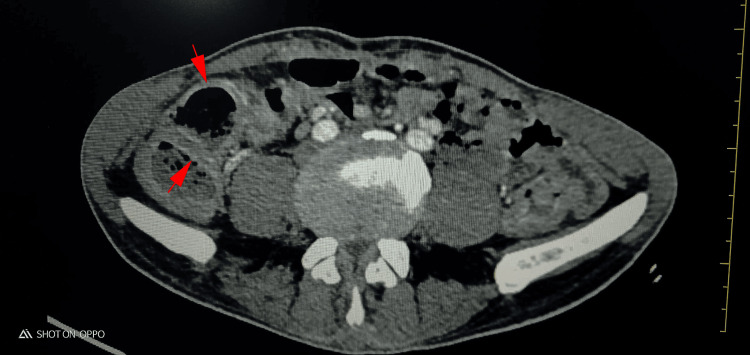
Abdominal computed tomography (CT) scan revealing diffuse parietal swelling (red arrow) and contrast intake in the mucosal layer No obvious abscesses or intestinal perforations were found.
